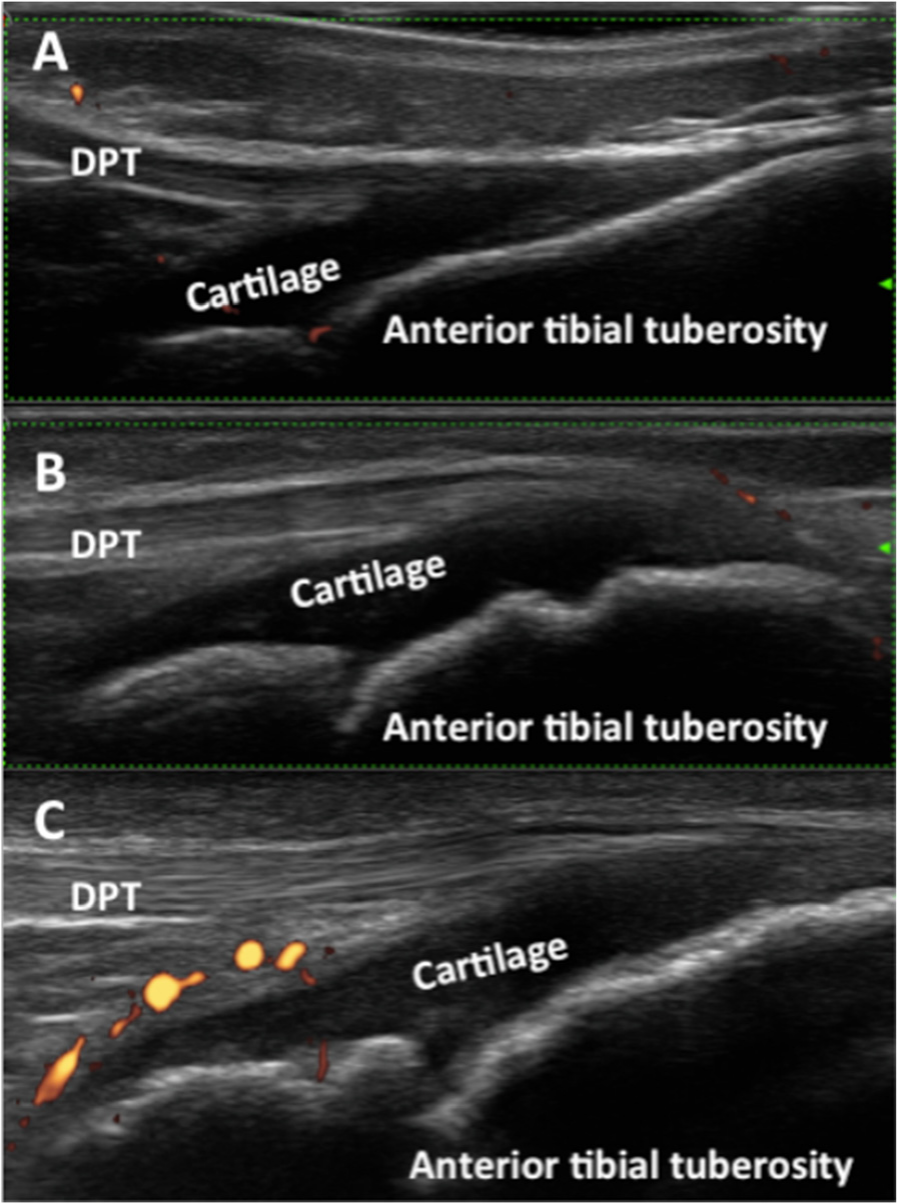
Sonographic appearance of the patellar enthesis at different ages. a. 2 years old girl; b. 8 years old girl; c. 10 years old boy. DPT: distal patellar tendon
